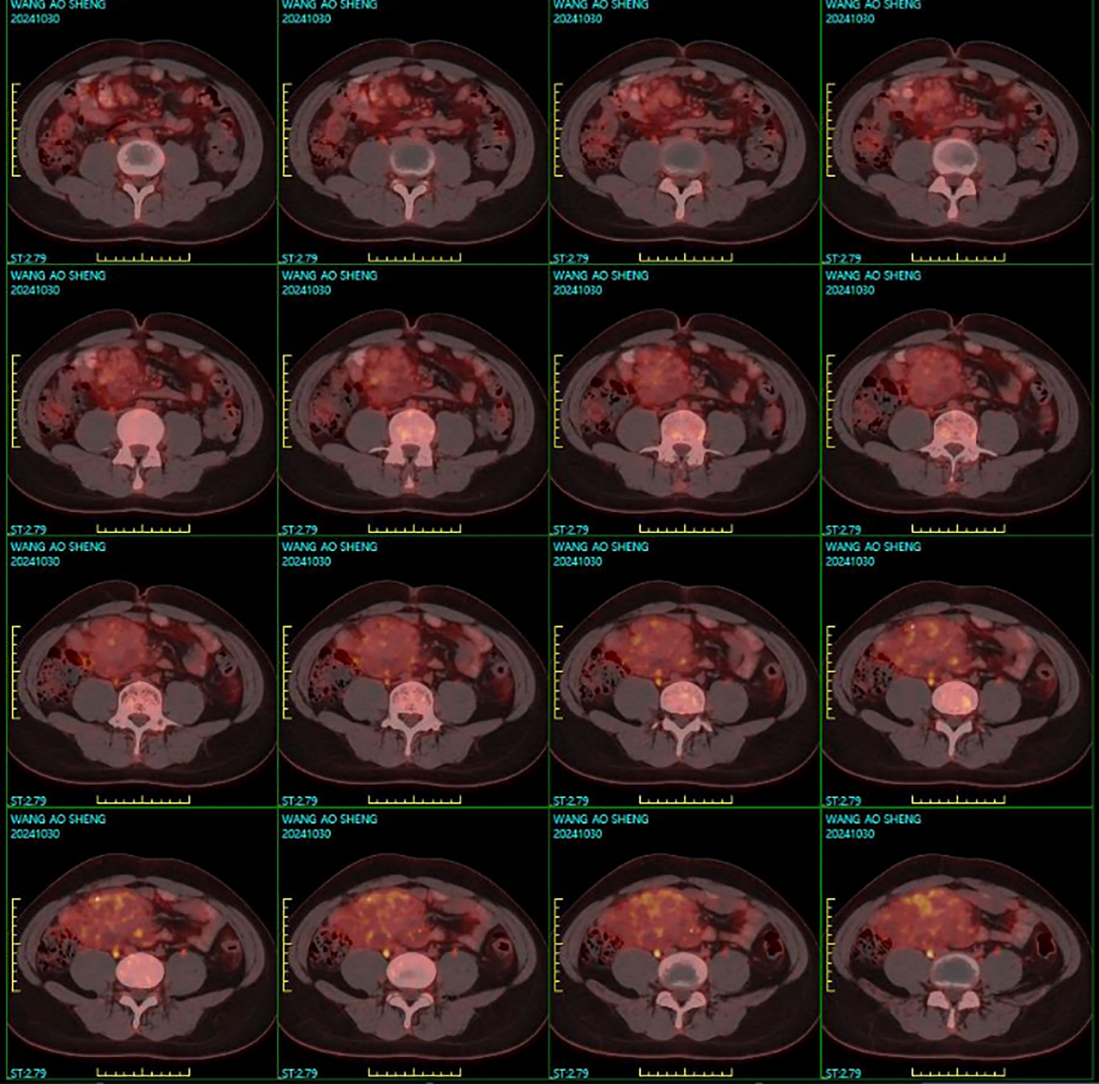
PET/CT imaging showing an irregular soft tissue mass in the mesenteric region of the right lower abdomen and pelvis. The lesion exhibits well-defined margins with mildly increased FDG uptake.
